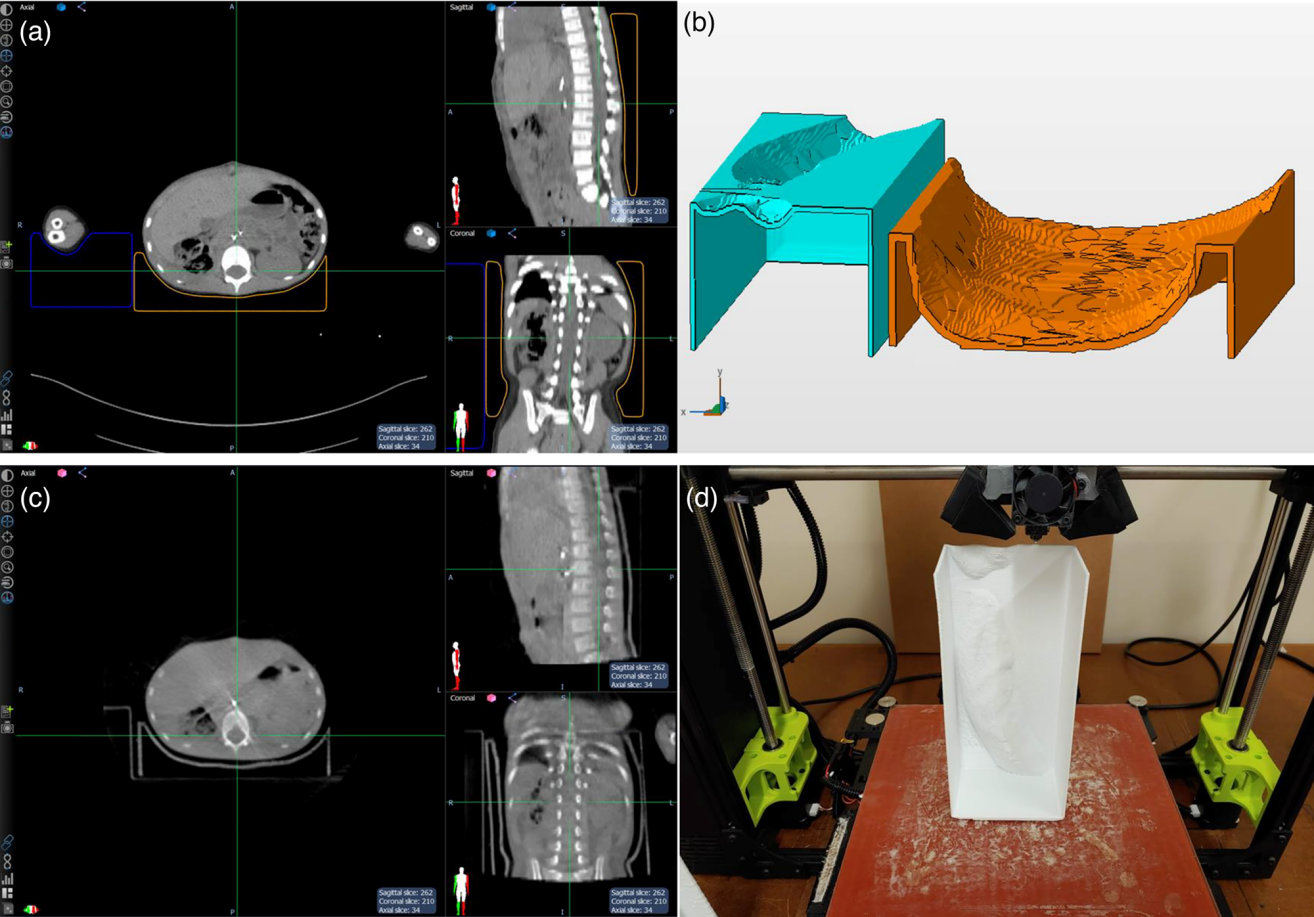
Generation of abdomen and arm immobilization 3D models, (a) axial, sagittal, and coronal view of the cubic structure subtracted from the 2 mm external body contour for the abdomen and arm, (b) 3D models for abdomen (orange) and arm (light blue) immobilization, (c) axial, sagittal, and coronal view of the daily CBCT, and (d) orientation of arm immobilization part on 3D printer.
